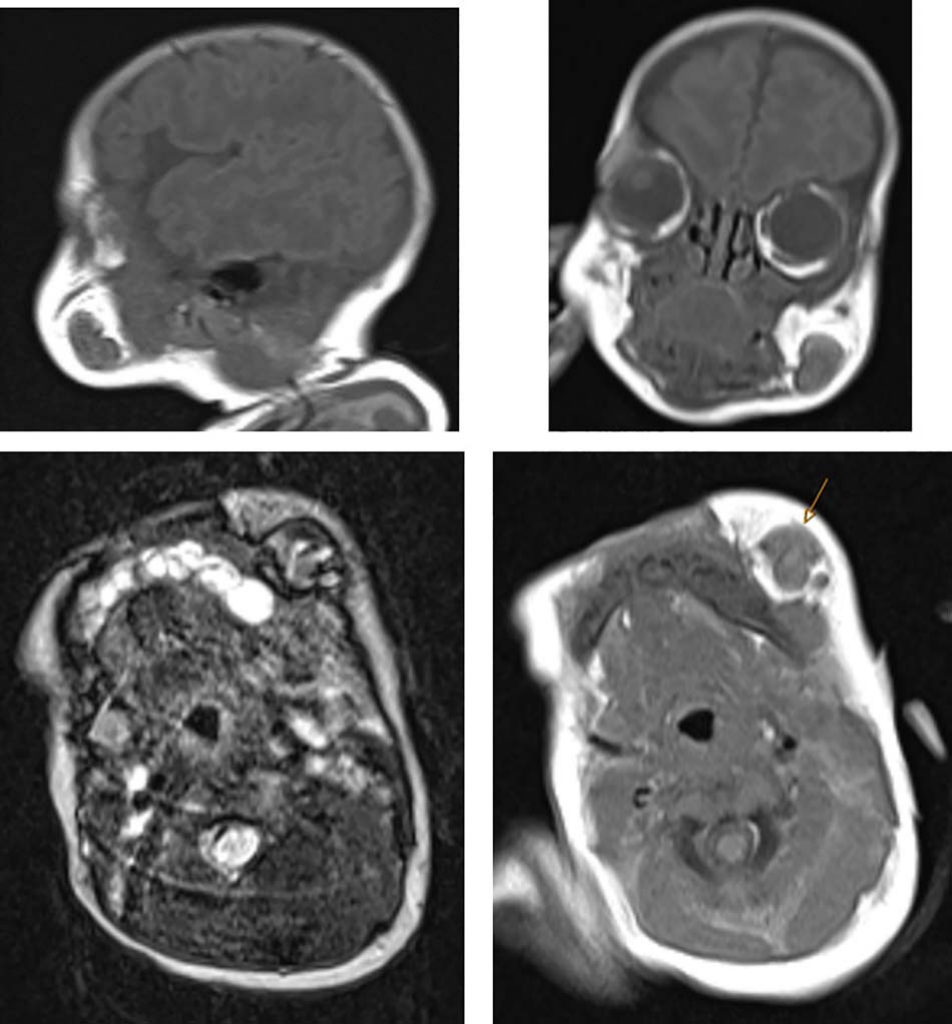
MRI showing the rupture of the parotid duct with venolymphatic malformation.
